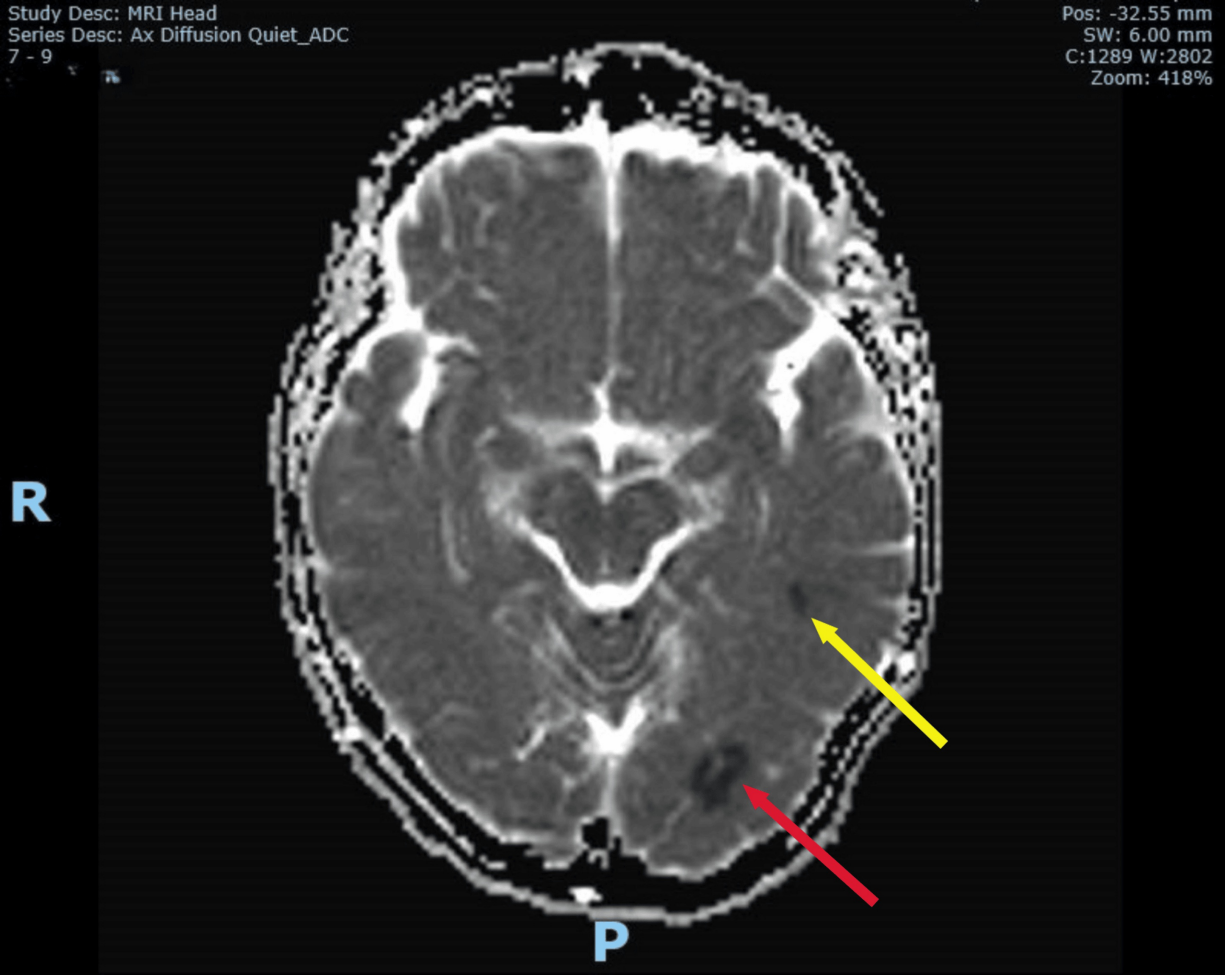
Corresponding lesions shown on MRI brain ADC confirming acute left posterior temporal (yellow arrow) and inferior occipital (red arrow) infarcts MRI: magnetic resonance imaging ADC: apparent diffusion coefficient
